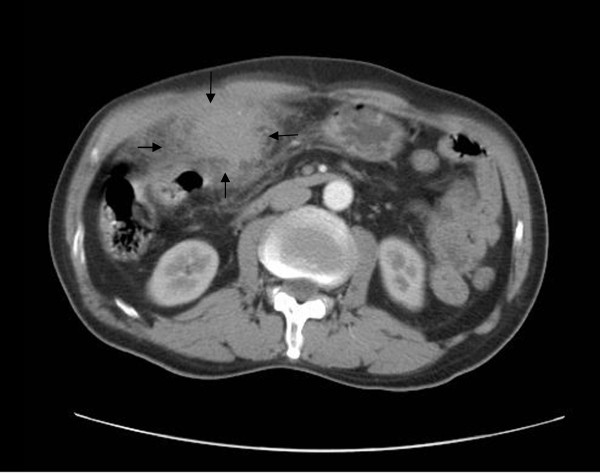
Abdominal computed tomography. Contrast-enhanced computed tomography during the arterial phase shows a hypodense lesion (arrows) in the left lobe of the liver, with infiltration to the surrounding tissue.
